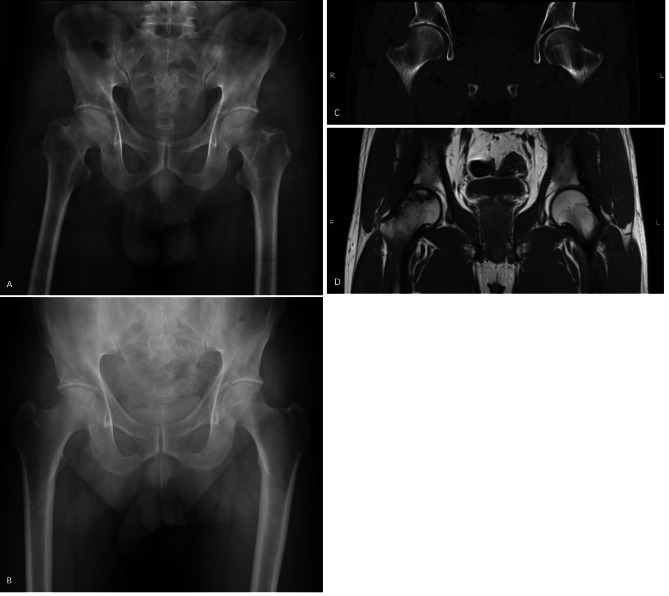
A 52-year-old male patient with ONFH of his right hip. He had undergone CD surgery 2 years previously. (A) Nevertheless, no evidence of a subchondral fracture, a fracture in the necrotic portion or flattening of the femoral head was observed. (B) Preoperative X-rays. (C) Preoperative CT. (D) Preoperative MRI.
